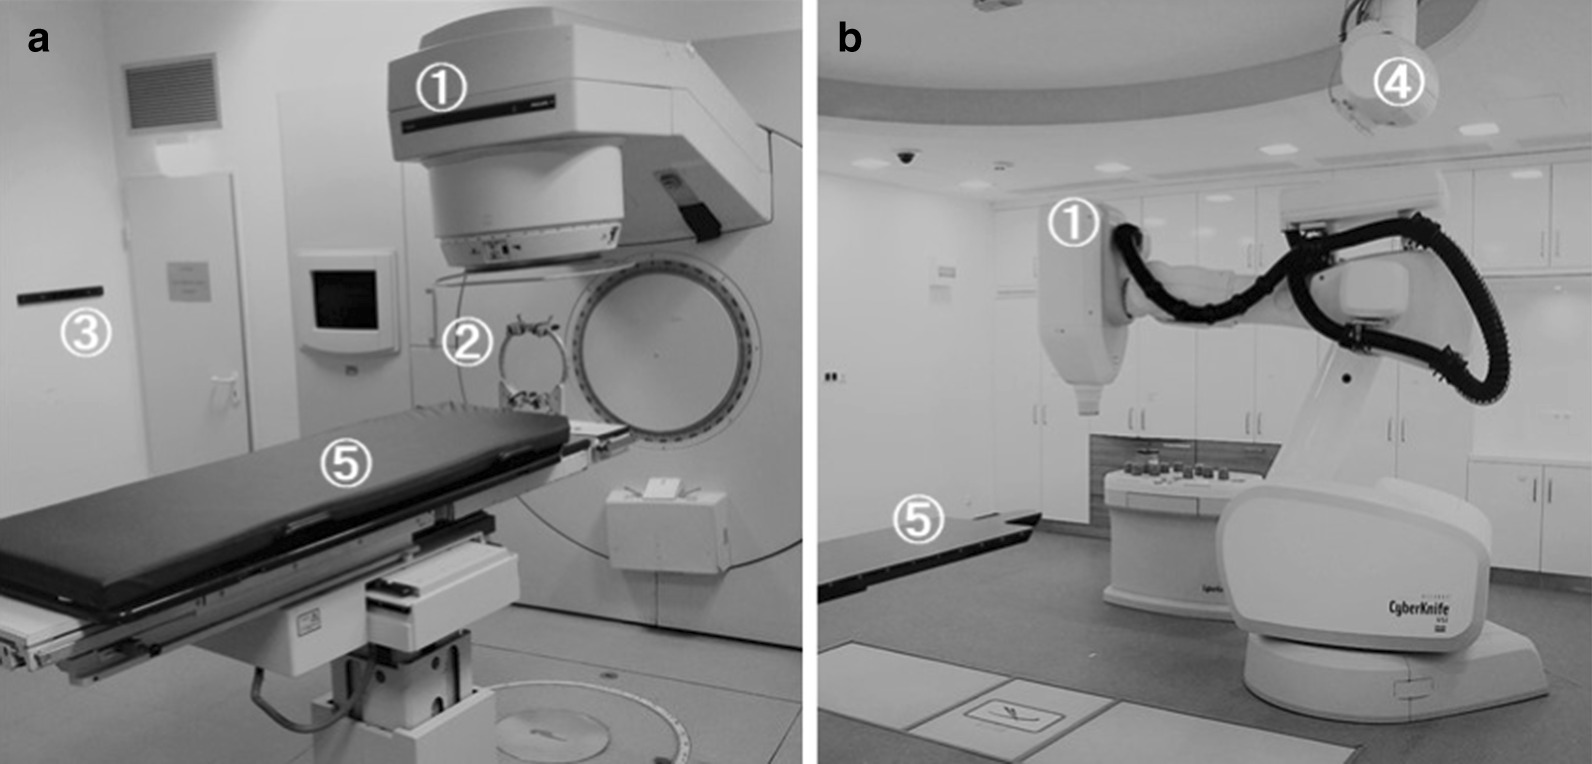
a Comparison of the LINAC (a) and the Cyberknife (b) setting. The relevant components are marked with numbers: (1) linear accelerator (2) stereotactic frame used in LINAC SRS for head fixation. (3) positioning laser (4) X-ray camera (5) mobile patient couch
